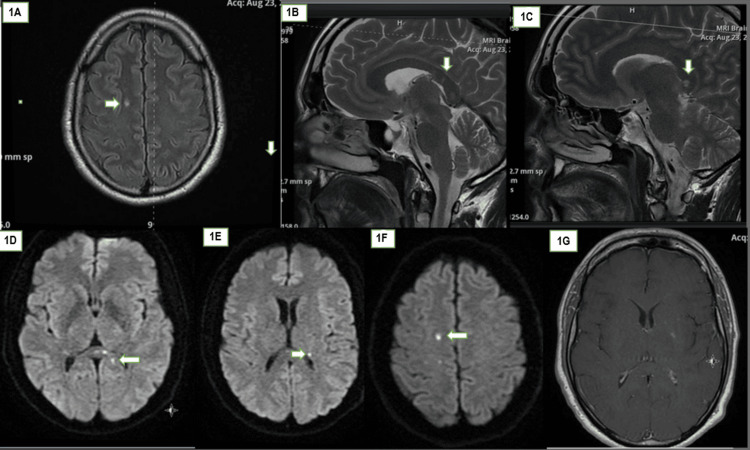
Brain MRI at the initial presentation in August 2020 (1A) A tiny FLAIR hyperintensity (arrow) in the deep white matter of the right hemisphere. (1B) A T2-weighted image showing a faint icicle lesion (arrow) in the posterior aspect of the corpus callosum. (1C) A T2-weighted image showing a small snowball lesion (a pathognomonic feature of Susac's syndrome) in the middle of the splenium of the corpus callosum. (1D) DWI with three tiny lesions in the splenium of the corpus callosum showing diffusion restriction. (1E and 1F) Other lesions showing diffusion restriction in the left and right hemisphere white matter, respectively. (1G) Post-contrast T1-weighted imaging showing a faint enhancement in the left internal capsule FLAIR: fluid-attenuated inversion recovery; DWI: diffusion-weighted imaging
